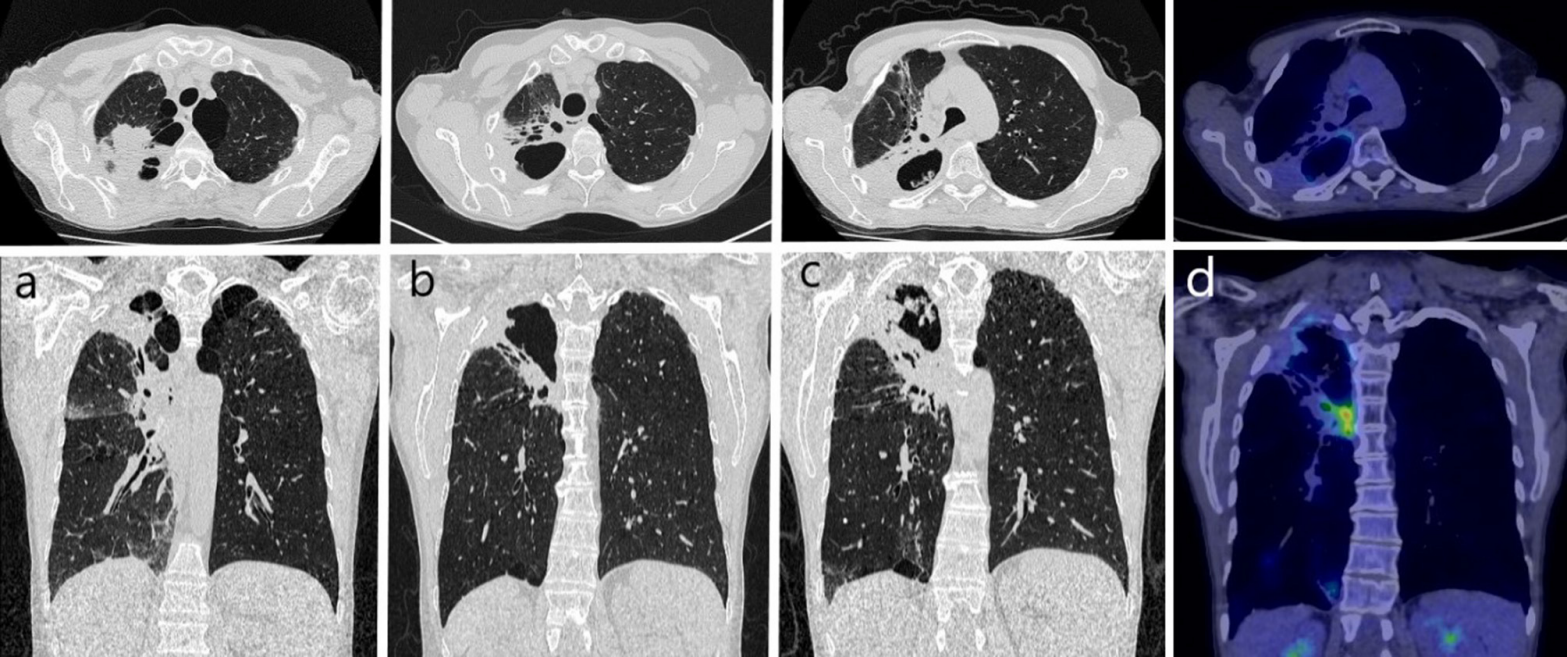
Chest CT in years 2016–2020: (a) 2016 - 3 months after chemoradiotherapy: solid tumor in the upper lobe of the right lung, emphysema in the apical parts of both lungs; (b) 2018 - cavity with the thick wall has formed at the site of the tumor in the right lung; (c) 2020 - progression of an irregular cavity in the upper part of the right lung with thick walls (concomitant mass on the posterior wall), surrounded by solid consolidation, dilated bronchus, and emphysema; (d) 2020 - positron emission tomography combined with computed tomography: unclear character of hypermetabolic masses in the right lung, more likely inflammatory, SUVmax = 2.5.
